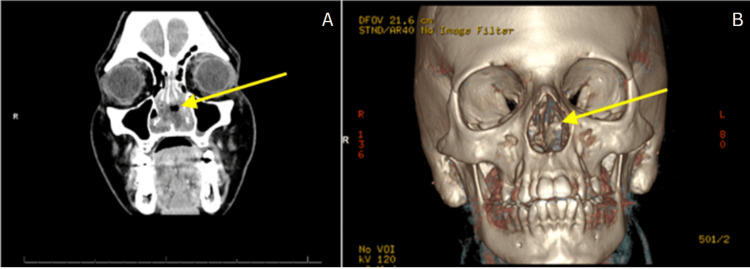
Sinus maxillofacial CT with contrast Figure 2A, per the radiology report, shows a nonspecific enhancing soft tissue within the nasal cavity associated with complete perforation of the nasal septum with overlying nasal soft tissue swelling and edema with no underlying abscess and no evidence of osteomyelitis of the underlying bone. Figure 2B is a 3D CT reconstruction demonstrating the same lesion.
